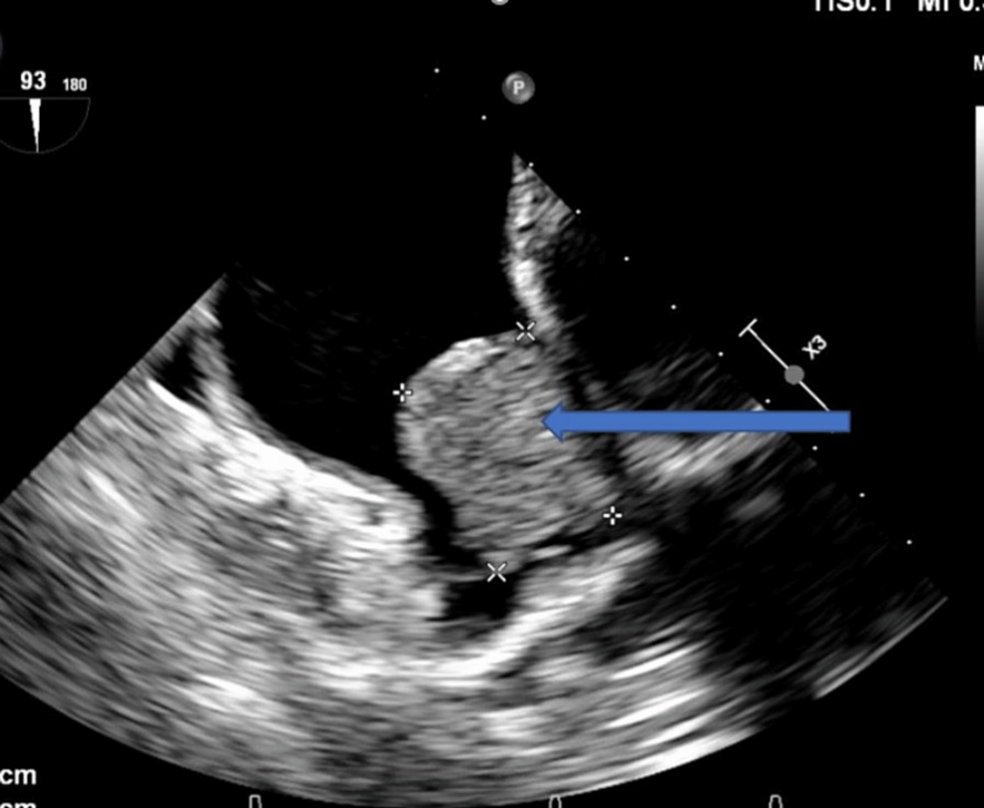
Transesophageal echocardiography showing myxoma attached to the left atrium (blue arrow).
